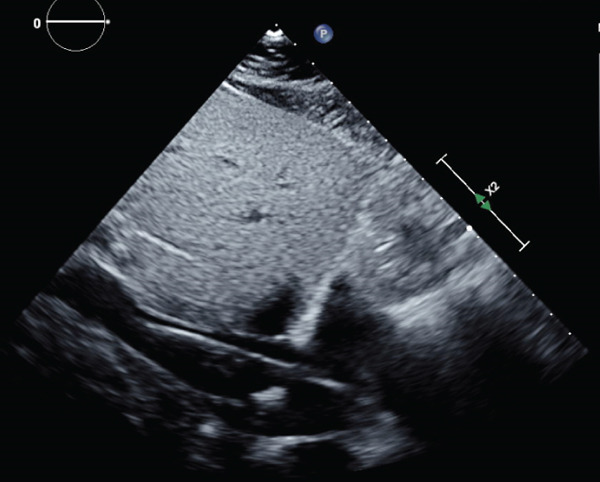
Subcostal view on transthoracic echocardiogram showing distal VV‐ECMO cannula in the inferior vena cava.
